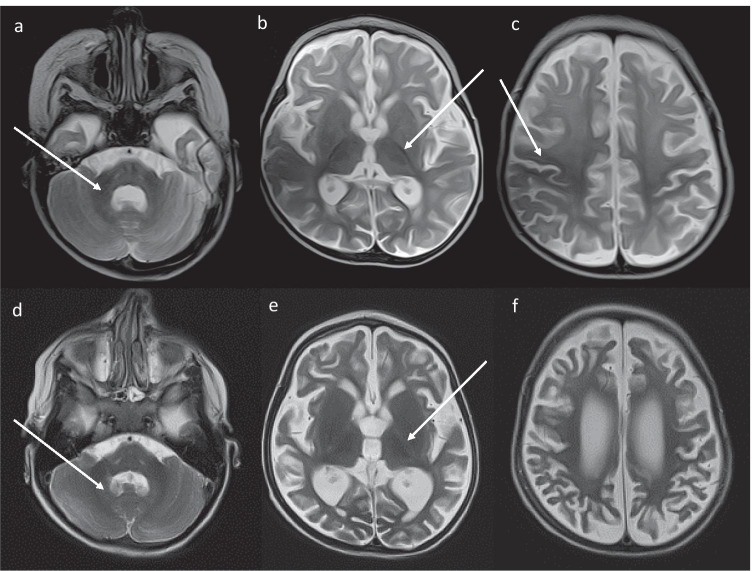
Upper row – MR brain examination at the age of 11 months, axial T2-weighted images. Hypointensity of the pons and cerebellar peduncles (a), posterior limbs of internal capsules (b), corpus callosum (not shown), perirolandic cortex (c) are visible, indicated myelination. Diffuse hyperintensity of the cerebral white matter of both hemispheres is seen. Mild enlargement of the ventricular system, enlargement of Sylvian fissures, subarachnoid spaces along the convexities and anterior interhemispheric falx are demostrated. Bottom row – MR brain examination at the age of 3 years, axial T2-weighted images. Hyperintensive signal of hilum of dentate nuclei (d) and posterior limbs of internal capsules (e) suggested degeneration of myelin. Progression of the atrophy of the cerebral hemispheres with severe cortical and subcortical atrophy (e, f)
